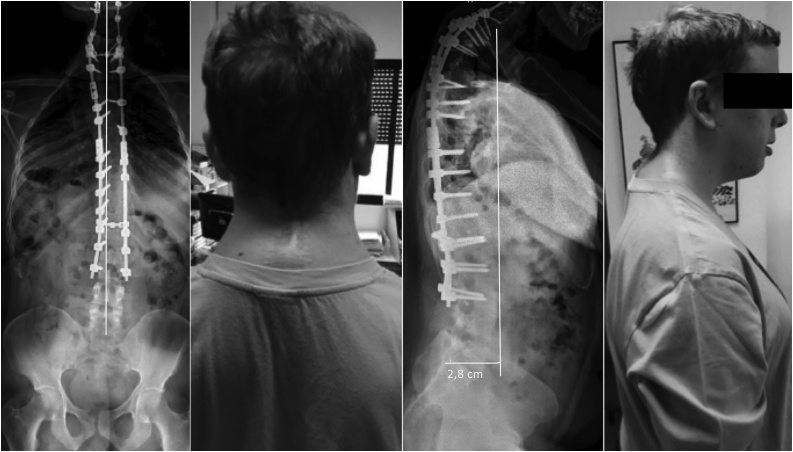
To prevent progression of deformity and complications we extended the upper instrumentation to cervical spine. By CT angiography, anomalous route of vertebral artery was ruled out and screws were placed at C2 pars interarticularis, lateral masses of C3–C6 lateral masses and C7 pedicle. A Ponte osteotomy at T1–T2 was performed to reduce kyphosis.
